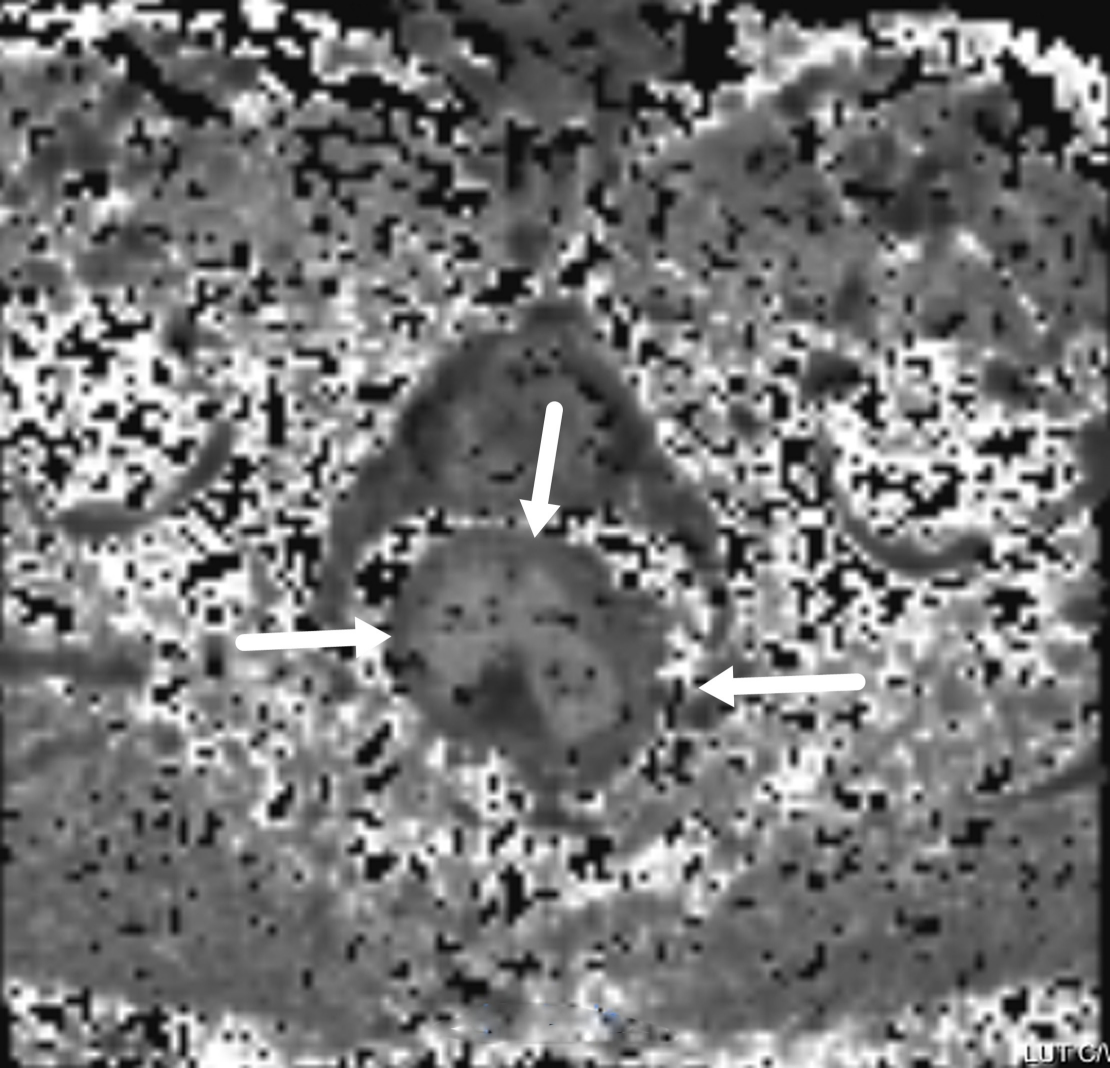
An MR image of a rectal carcinoma. The MK map shows unevenly high signals.
